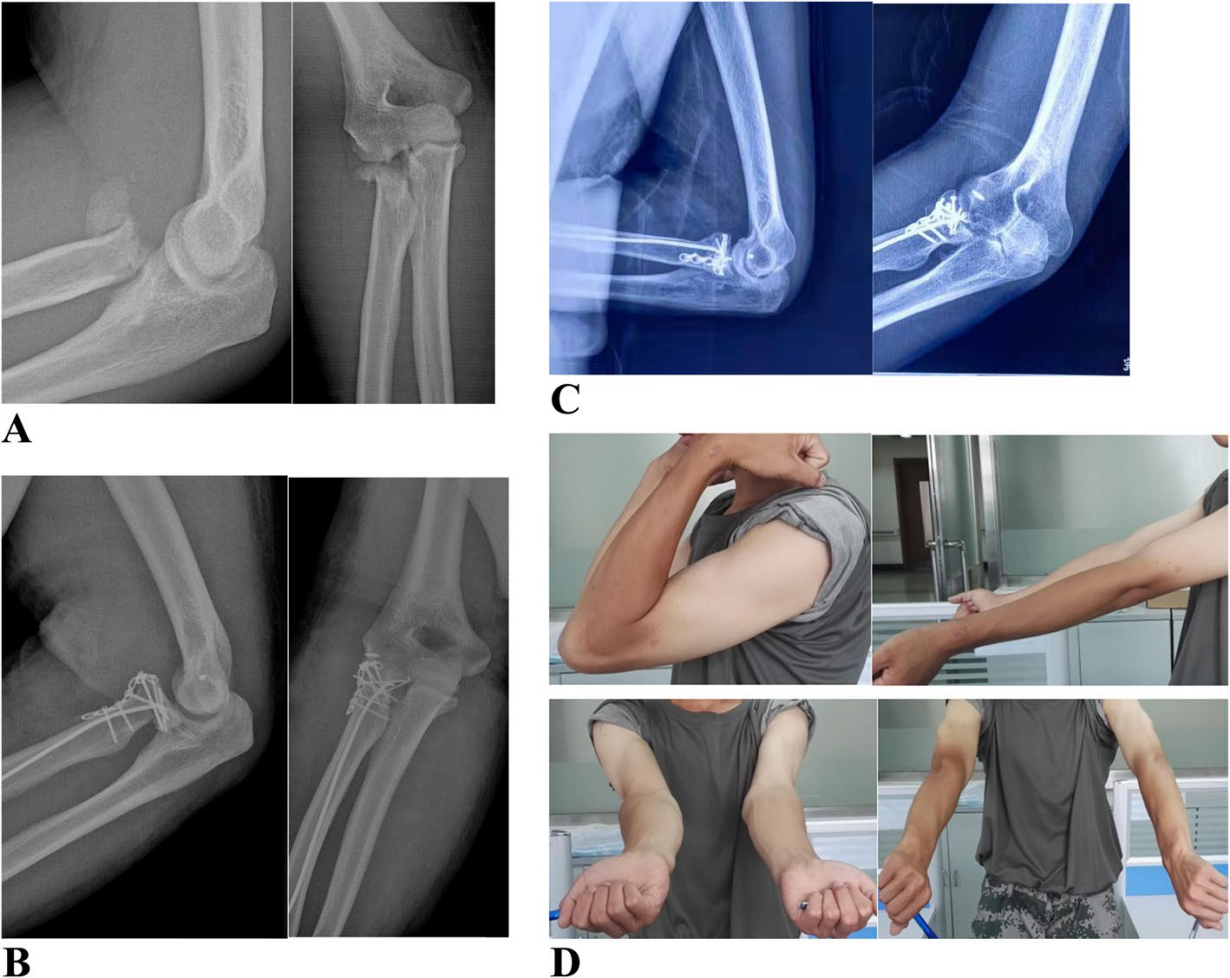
Typical case: left radial head fracture. a Preoperative radiograph showed Mason type III radial head fracture. b Postoperative radiograph revealed a good restoration of alignment between the radial head and neck. c Anteroposterior and lateral radiograph showed bone union at 12 months after surgery. d Functional outcomes at the final follow-up. Compared with the unaffected sides, the ROM of elbow and forearm rotation was sufficient
